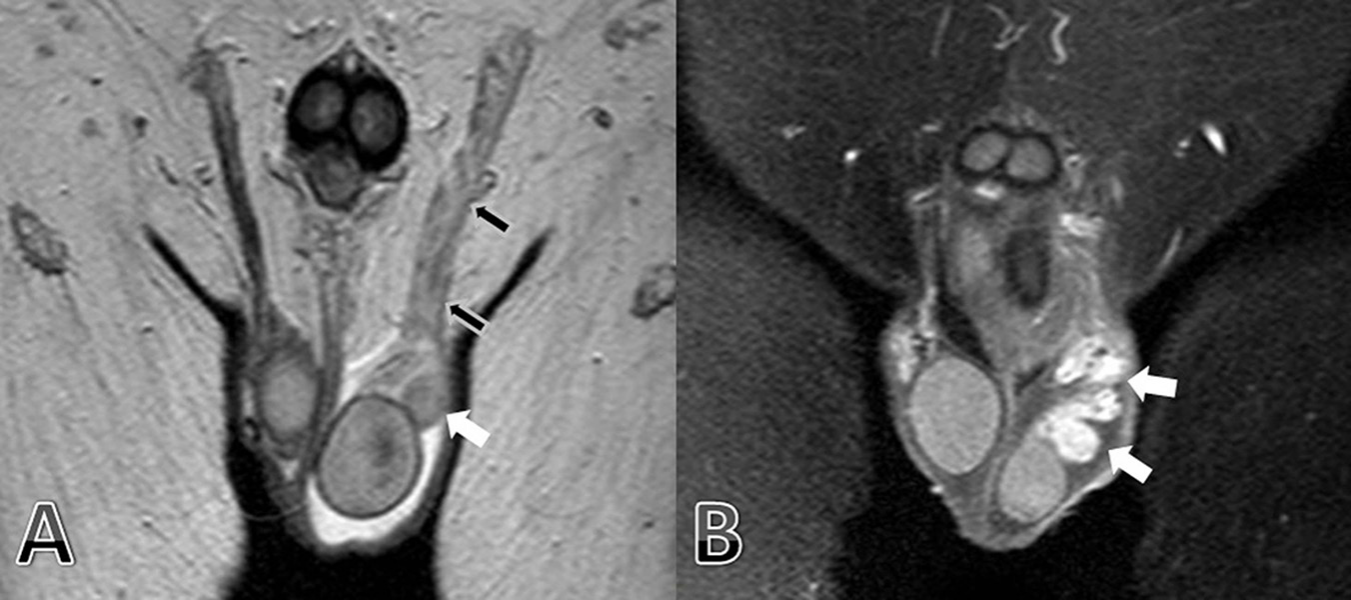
Coronal T2 Weighted (a) MR Imaging Shows Marked Edema of the Left Epididymis (Head-tail and Body, White Arrows) and Ductus Deferens (Black Arrows). After Contrast Media Administration on Coronal T1-weighted (b) MR Imaging Shows Marked Enhancement of the Left Epididymis (Head-Tail and Body, White Arrows).
